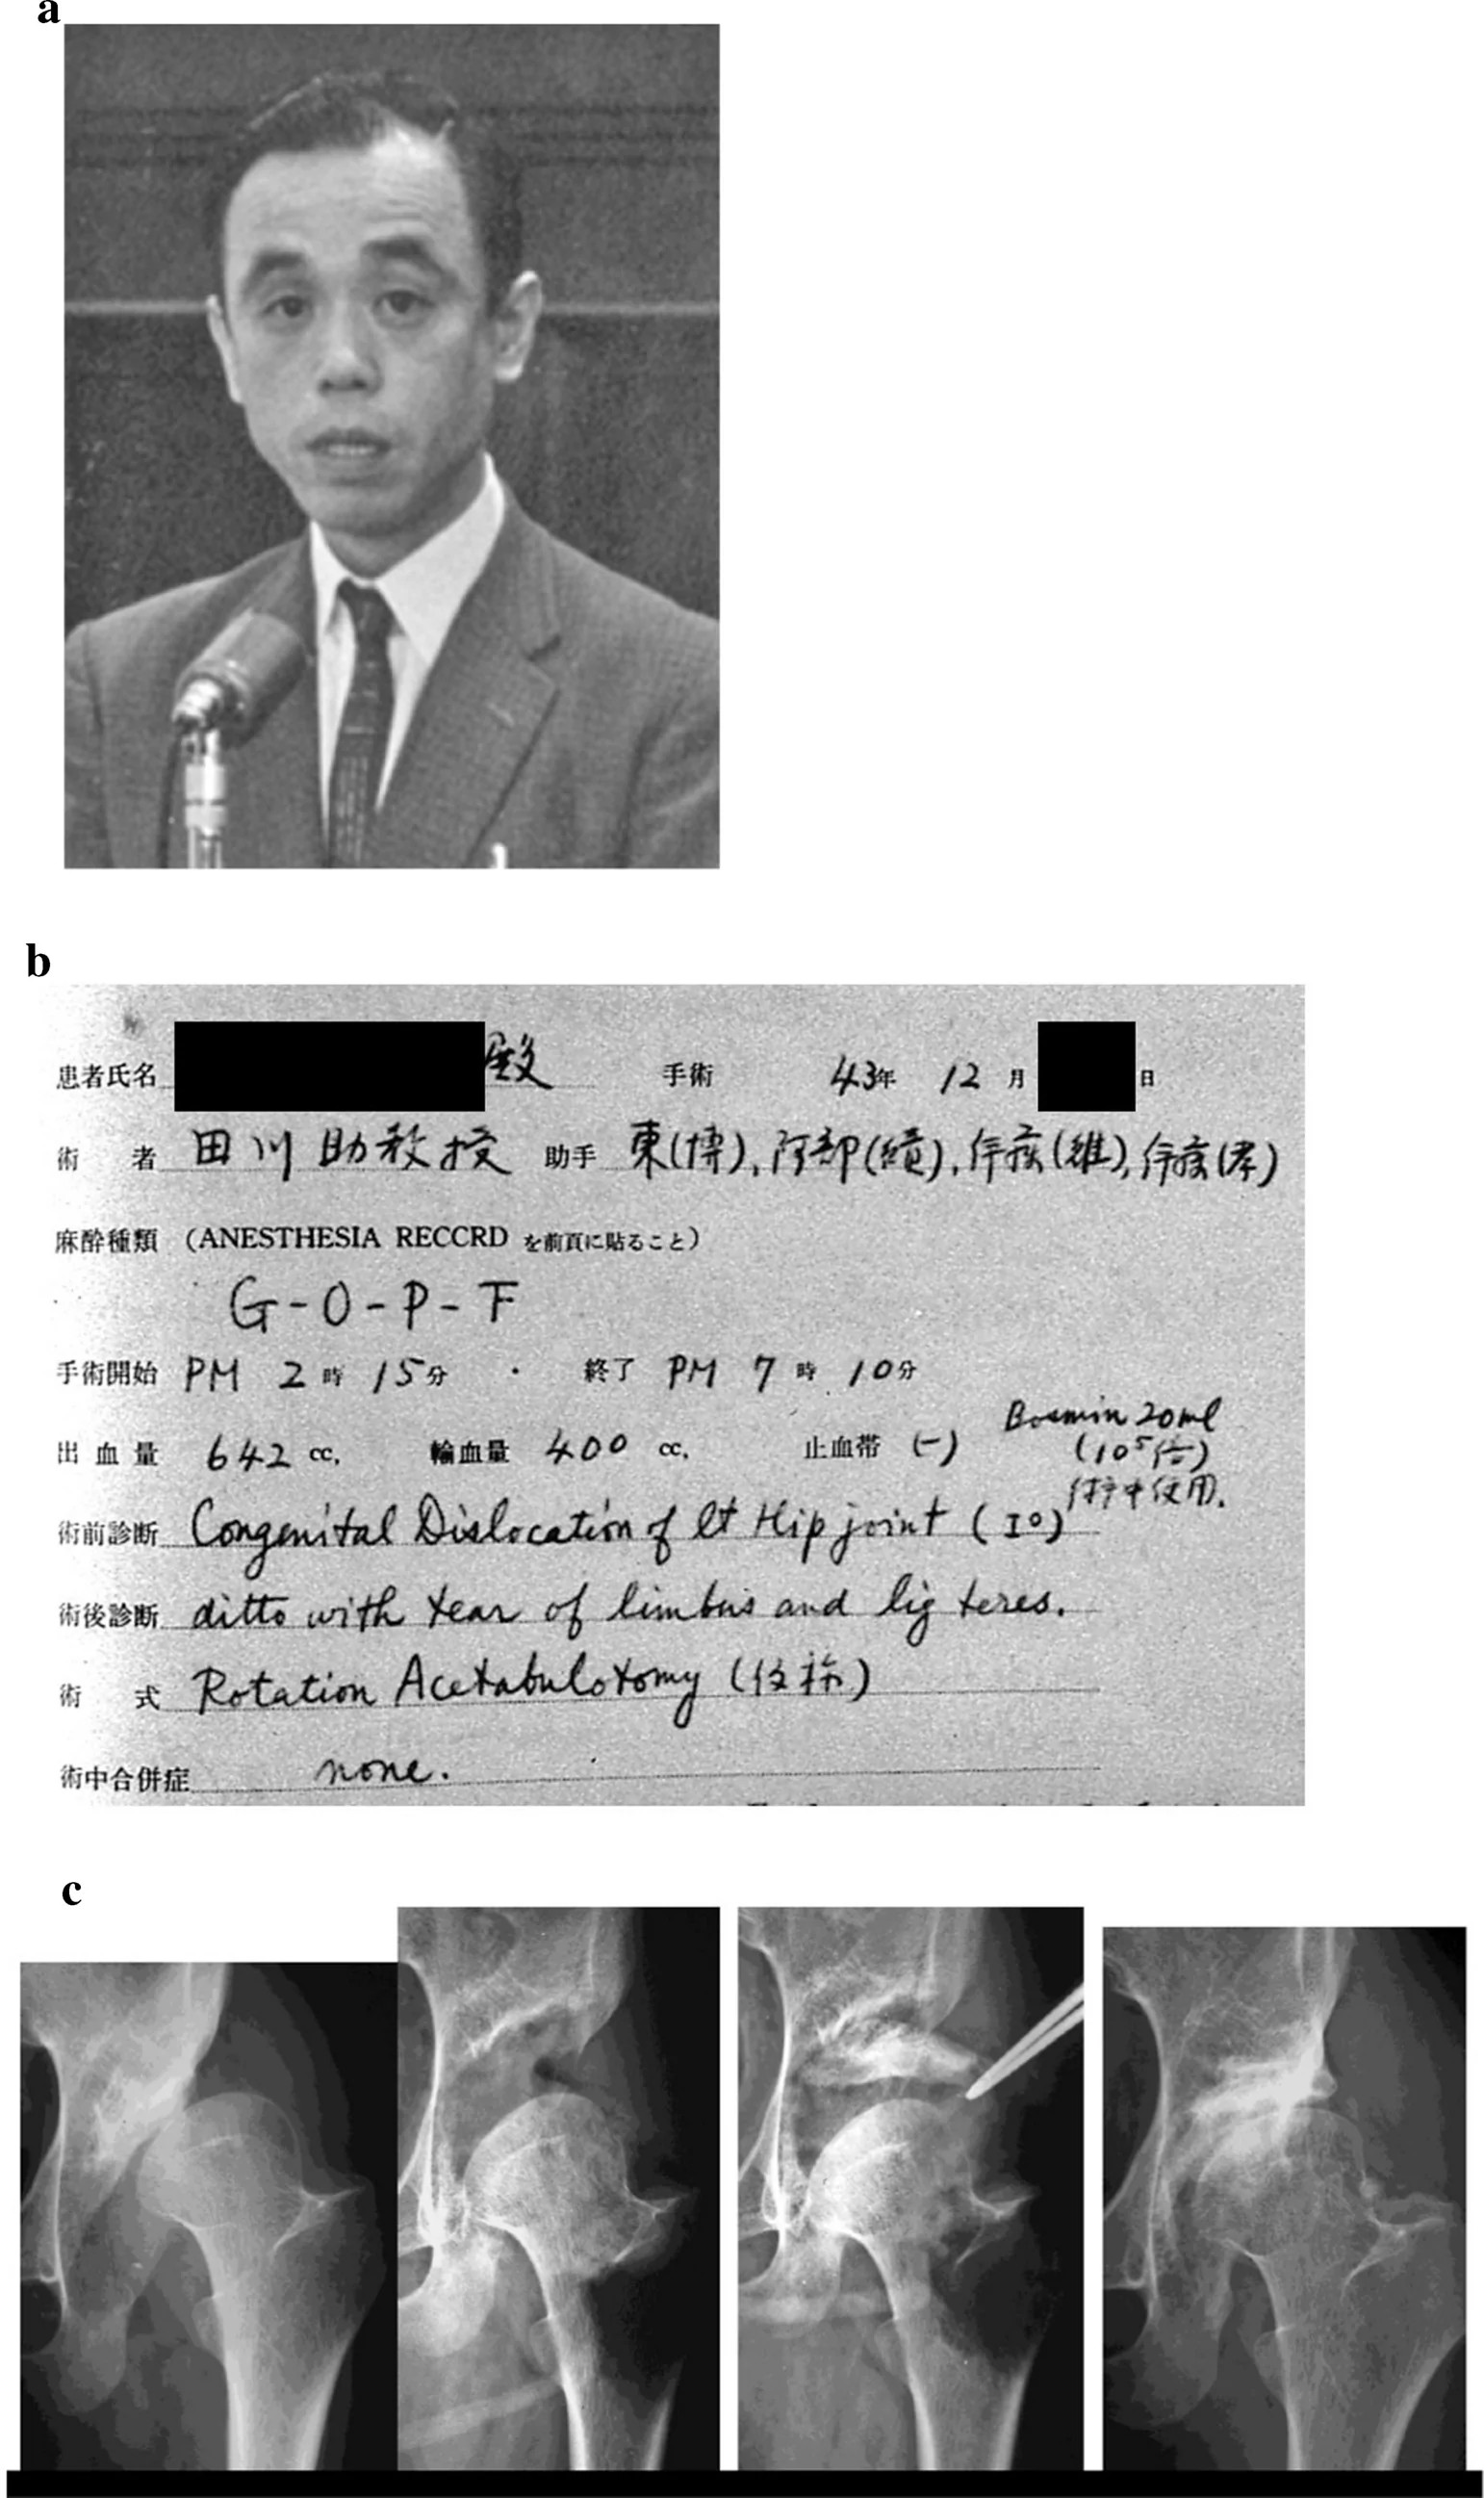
a Dr. Hiroshi Tagawa, who established rotational acetabular osteotomy (RAO). b Original operative record of the first RAO performed in 1968. c Serial anteroposterior radiographs of the first patient treated with RAO: preoperative, intraoperative, immediate postoperative, and 4 months postoperatively
